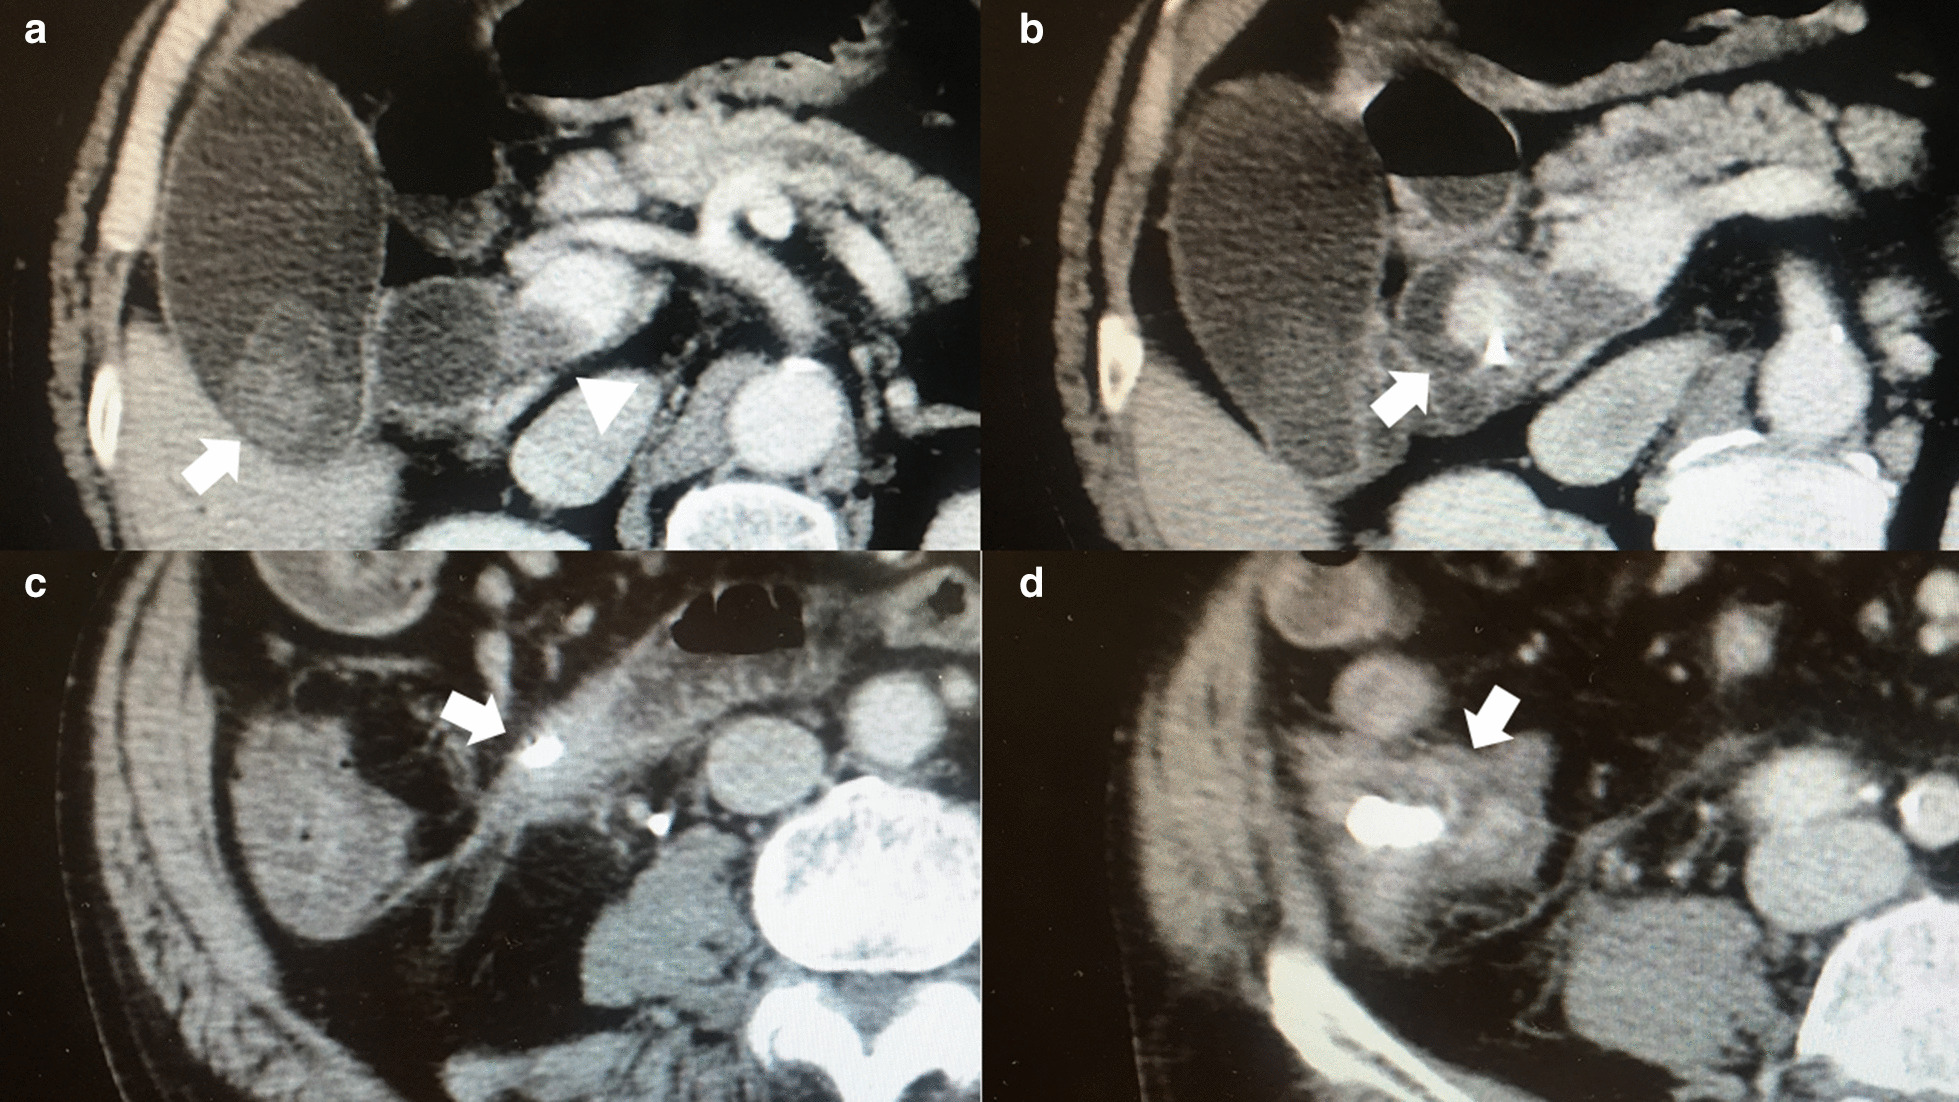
Findings of preoperative abdominal contrast-enhanced computed tomography regarding the choledocholithiasis and the endoscopically inserted biliary drainage tube. Several gallstones existed in the gallbladder and common bile duct (CBD). Maximum stone with 3cm in diameter was observed in CBD (White arrows). Furthermore, distal end of the endoscopically inserted biliary drainage tube penetrated through the duodenum to the cecum (White arrowheads). (*Ce* cecum; and *Du* duodenum)
